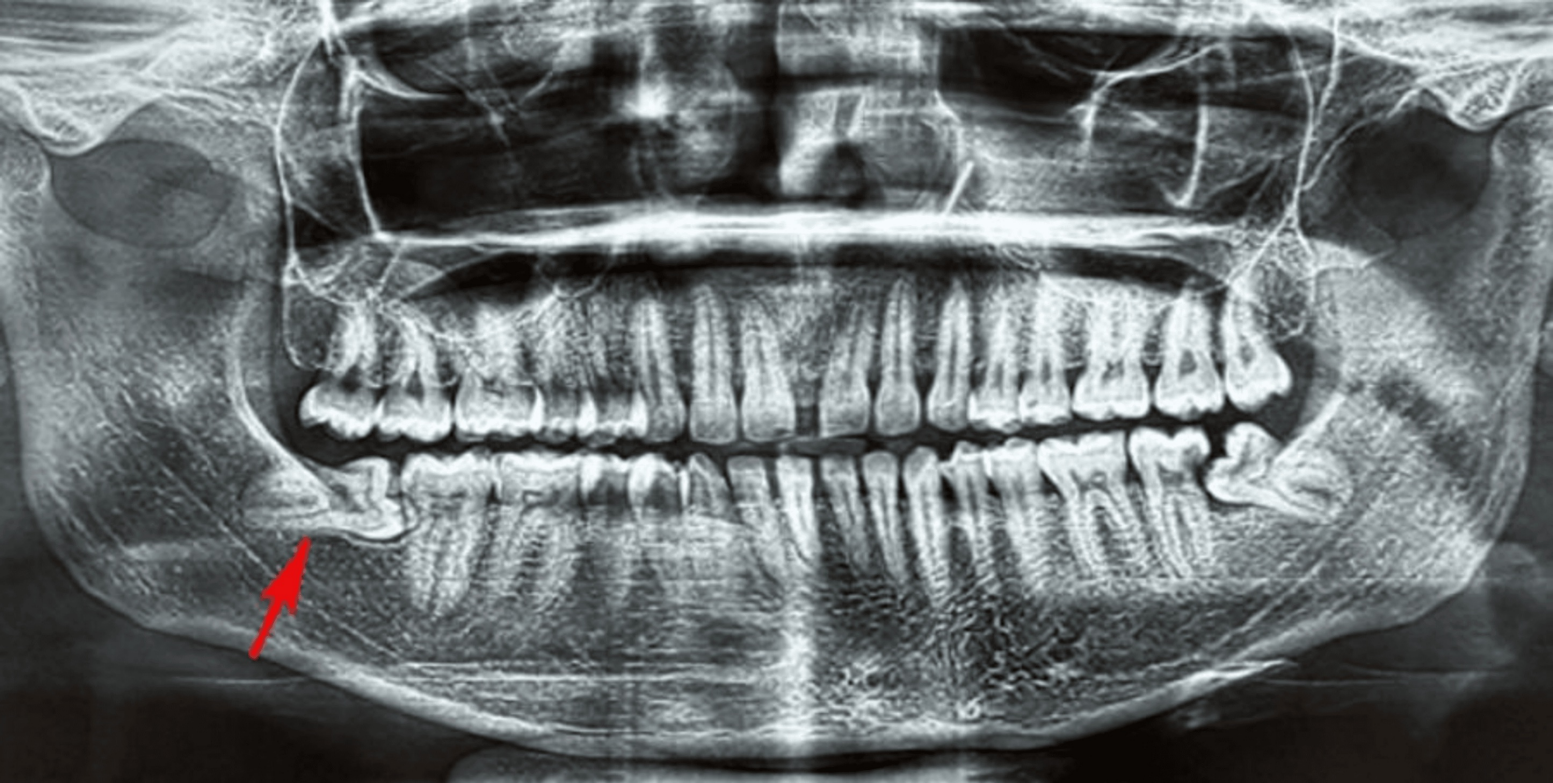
Orthopantomogram showing an impacted mandibular third molar tooth on the right side (red arrow).
